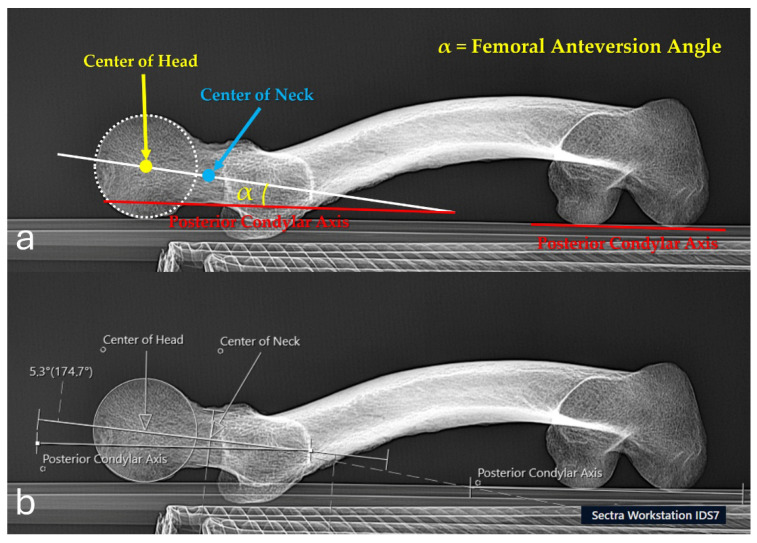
Measurement of femoral neck anteversion on modified Budin radiographs. (a) Demonstration of the measurement technique on a modified Budin view: the centers of the femoral head (yellow) and neck (blue) are identified, the line connecting these points represents the femoral head–neck axis, and the posterior condylar axis is drawn along the most posterior aspects of the femoral condyles. The angle α between the femoral head–neck axis and the posterior condylar axis is defined as the femoral neck anteversion angle. (b) The same measurement performed on the digital radiography workstation (Sectra IDS 7), showing the angle calculation between the femoral head–neck axis and the posterior condylar axis.
